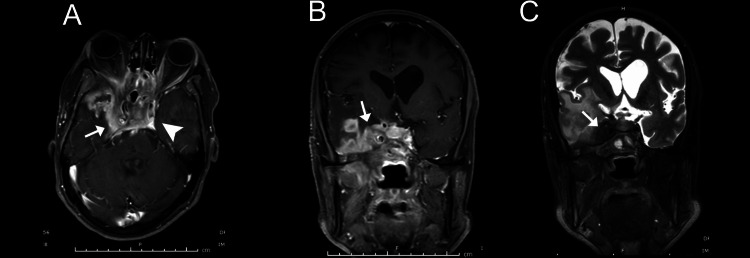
A. An axial T1-weighted image (post-contrast) showing an enhancing soft tissue lesion involving the right cavernous sinus and adjacent temporal region. The left cavernous sinus is normal (arrowhead); B. A coronal T1-weighted image (post-contrast) shows enhancing soft tissue involving the right cavernous sinus and encasing the cavernous segment of the right internal carotid artery (ICA) and adjacent temporal region (arrow); C. A coronal T2-weighted image showing a hypointense lesion involving the right cavernous sinus encasing the right ICA (arrow).
